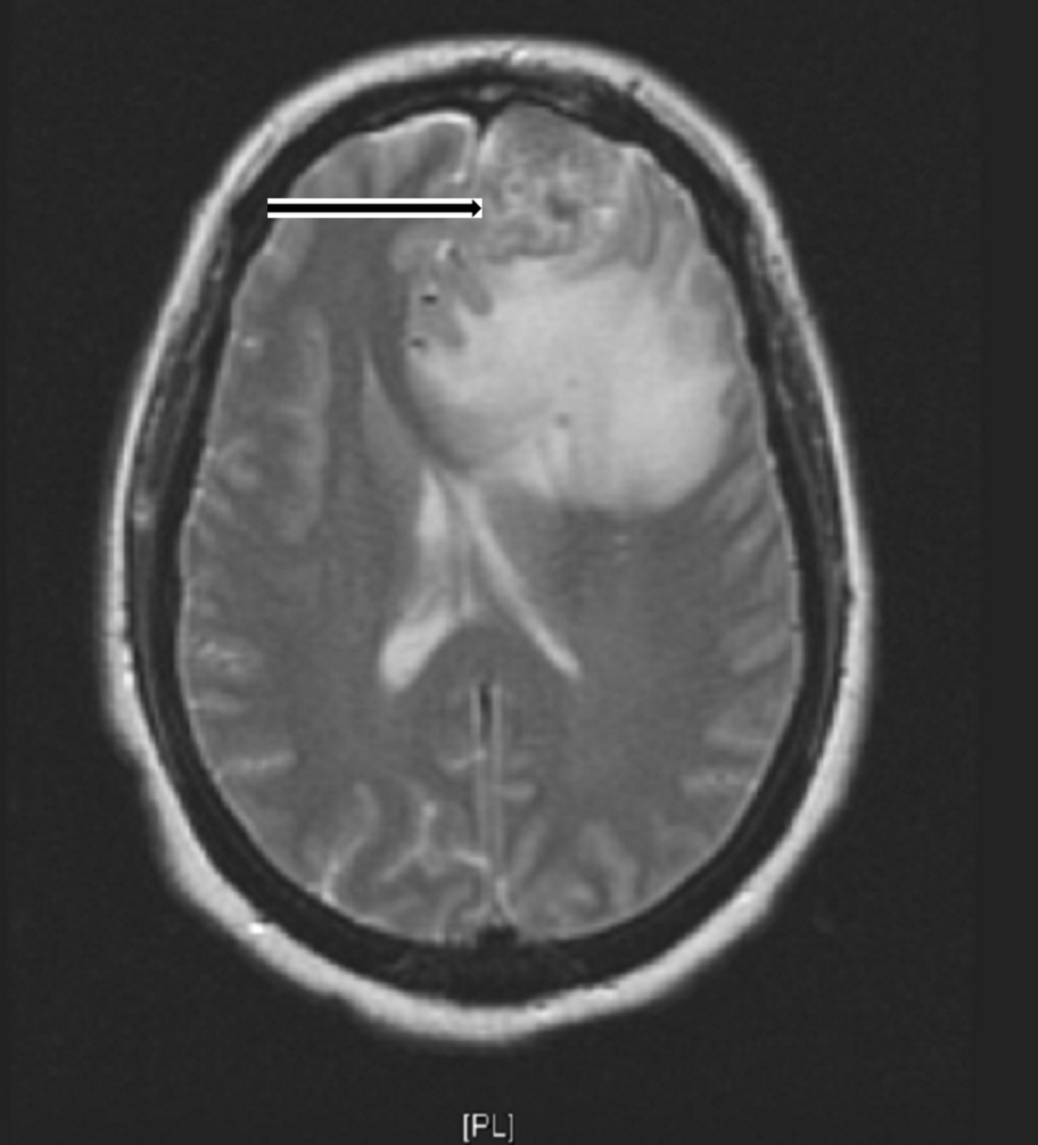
T2 Weighted MRI brain showing left frontal mass (black arrow) with surrounding vasogenic edema and midline shift MRI: magnetic resonance imaging
